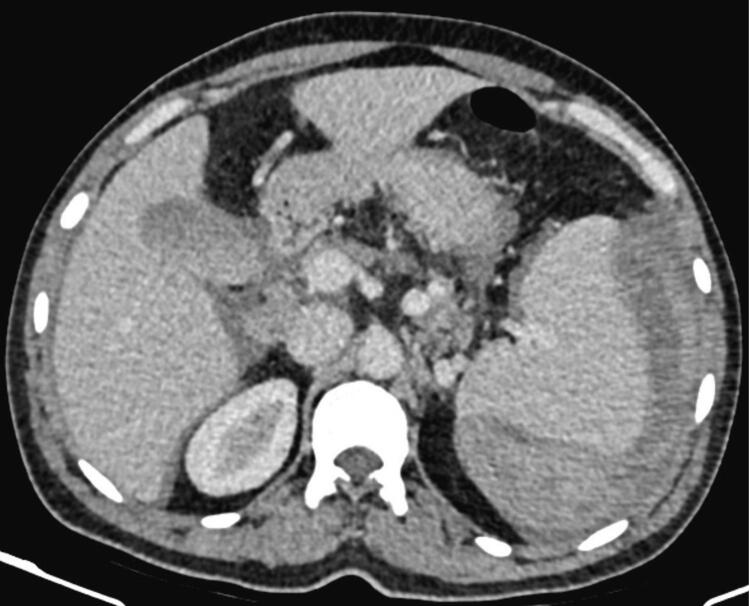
Contrast-enhanced computed tomography (CECT) of the abdomen, arterial phase (axial view) revealing splenic contrast blush.
